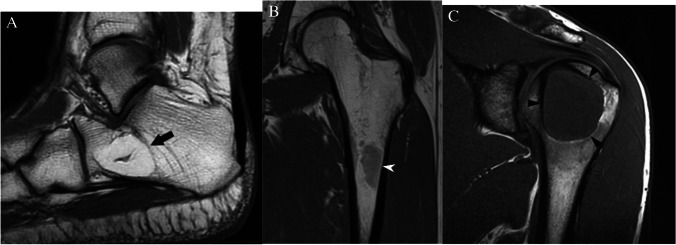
MRI lesions based on T1 signal intensity. T1-weighted MRI images show A a calcaneal intraosseous lipoma (arrow) that has signal intensity much higher than skeletal muscle with the same signal intensity as fat, B a focus of red marrow (arrowhead) in the proximal femur that is slightly hyperintense to skeletal muscle, and C a chondroblastoma (arrowheads) in the humeral that has signal isointense to skeletal muscle
